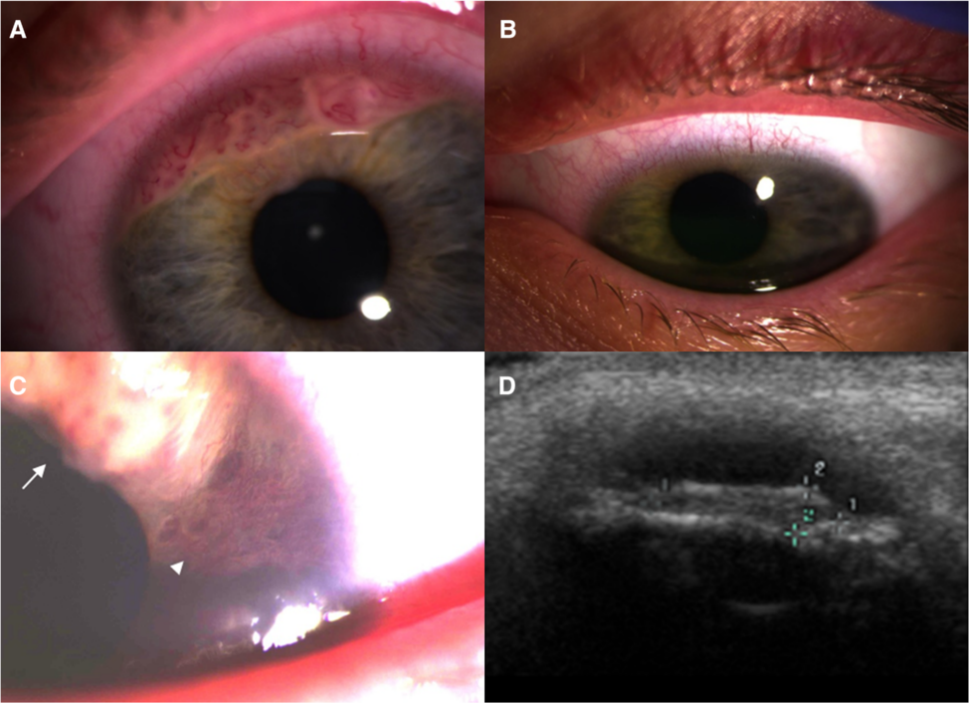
Left eye iris mass at presentation (a), resolved after initial systemic chemotherapy (b). Ocular tumour relapse (*arrow*) and neovascularization of the iris (*arrowhead*) (c), demonstrated also on B mode ultrasound scan (d)
